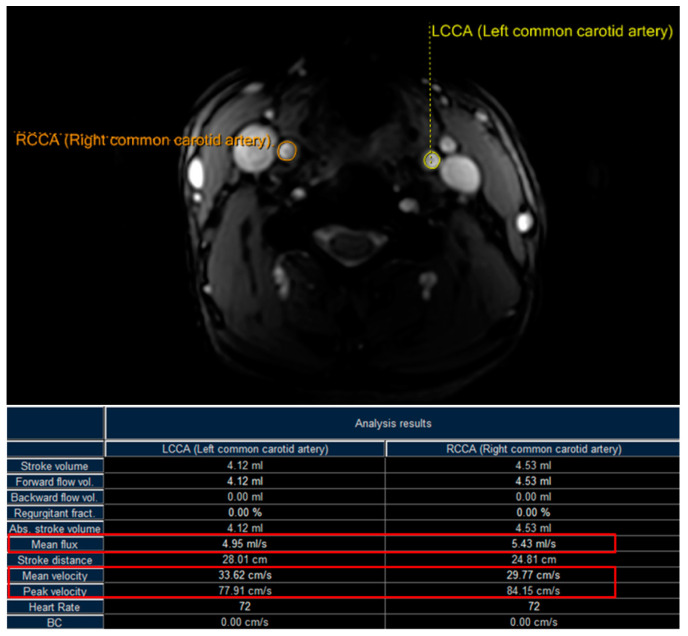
PC-MRI measurements of both the left and right common carotid arteries for the maximum and time-averaged mean velocities, and volume flow rate (“Peak velocity”, “Mean velocity”, and “Mean flux” denoted in the figure).
